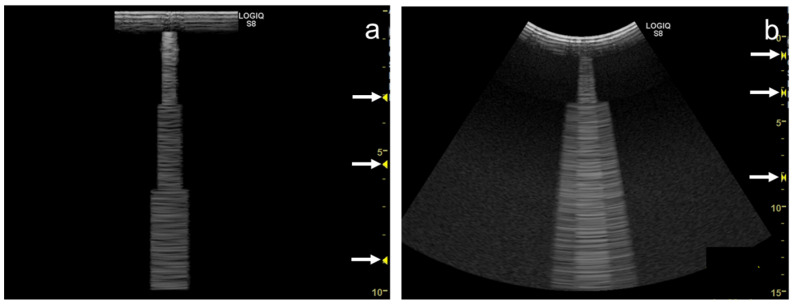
Ultrasound images obtained by a linear transducer (a) and a curvilinear transducer (b) with multiple focus (arrows), which causes step-wise configuration in a vertical artifact.
